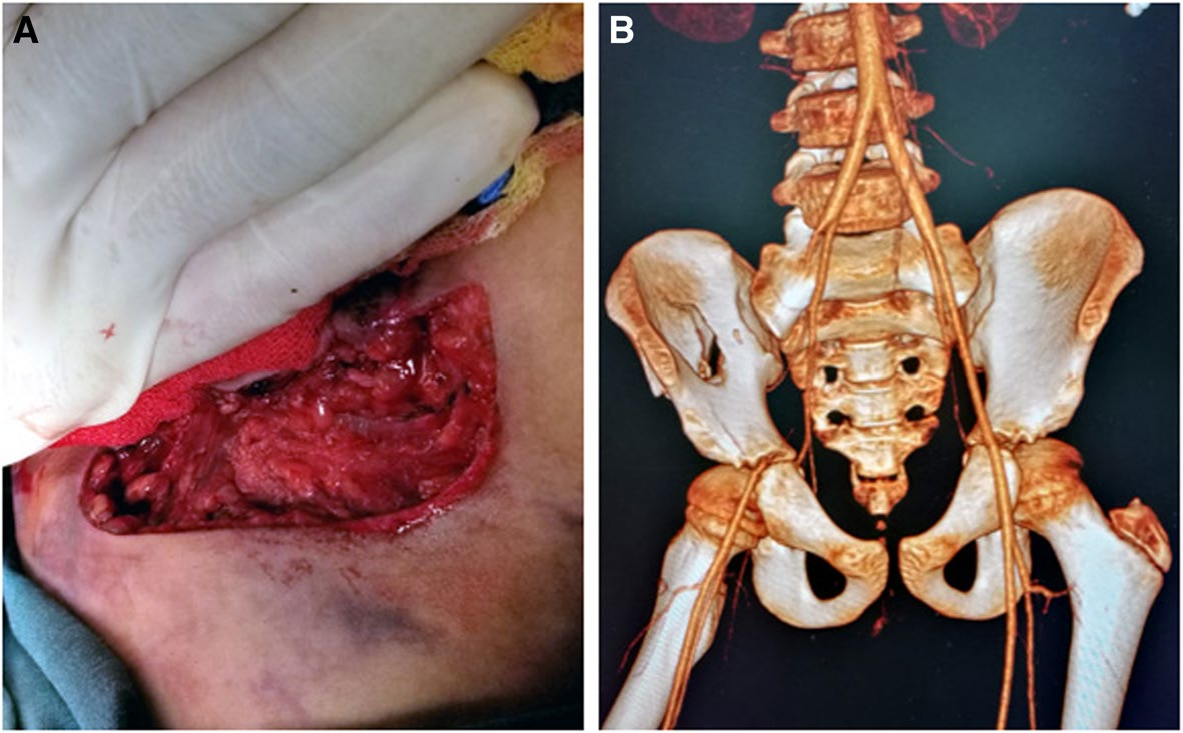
A An irregular wound approximately 12 cm in length in the right groin, which is under external manual compression. B CT scan detected fracture of right ilium wing, EIA was tapered to be completely transected. The common femoral artery was redeveloped through branches of internal iliac artery
